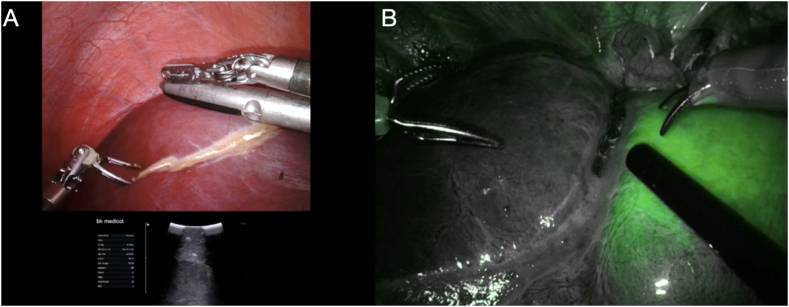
A. Use of Intraoperative UltraSound to identify metastases margins; B. Vascularization assessment during robotic liver resection after intravenous Indocyanine Green (ICG) injection.
